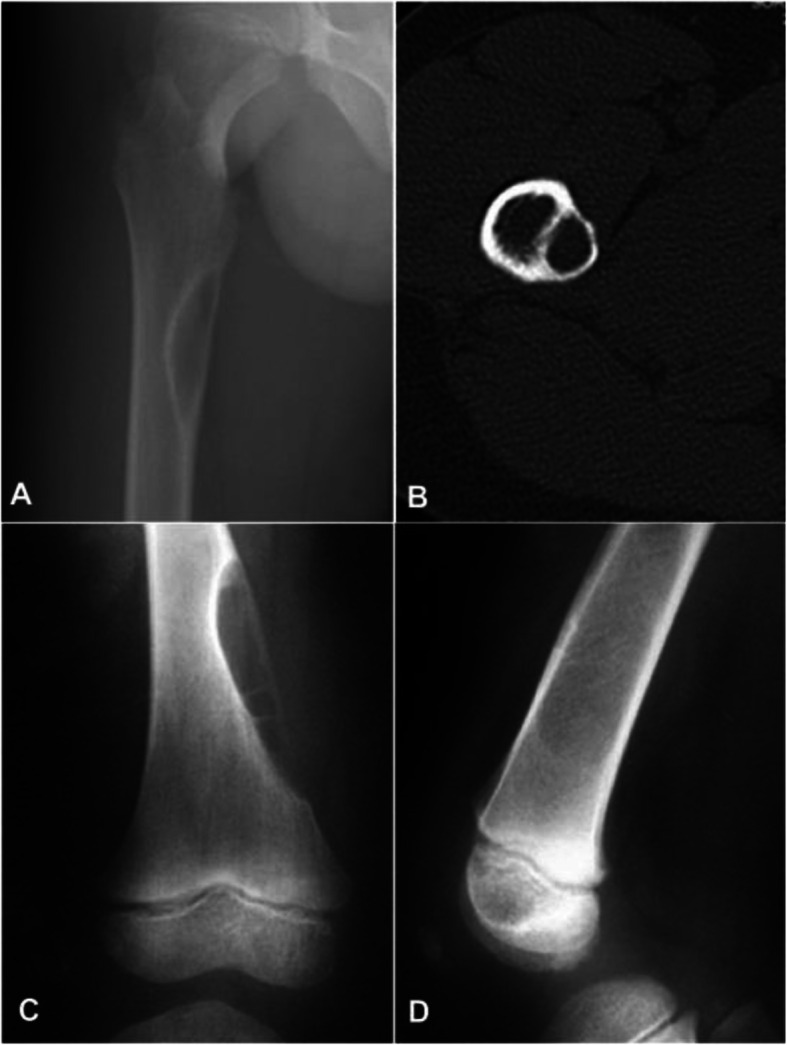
Imaging of intracortical hemangioma. a & b. Plain radiograph of the anteroposterior view of the proximal femur (a) and computed tomography imaging in transverse view (b) demonstrated an intracortical hemangioma lesion below the right femoral trochanter. The lesion was fusiform with the long axis parallel to the long axis of the femur and was expansile and osteolytic with osteodermic sclerosis at the edge. c & d. Plain radiographs of the anteroposterior (c) and lateral view (d) of the distal femur revealed an osteolytic bone defect with septa inside the lesion at the distal interior end of the left femur. The medullary side of the lesion was osteoslerotic with discontinuation of the interior bone cortex
